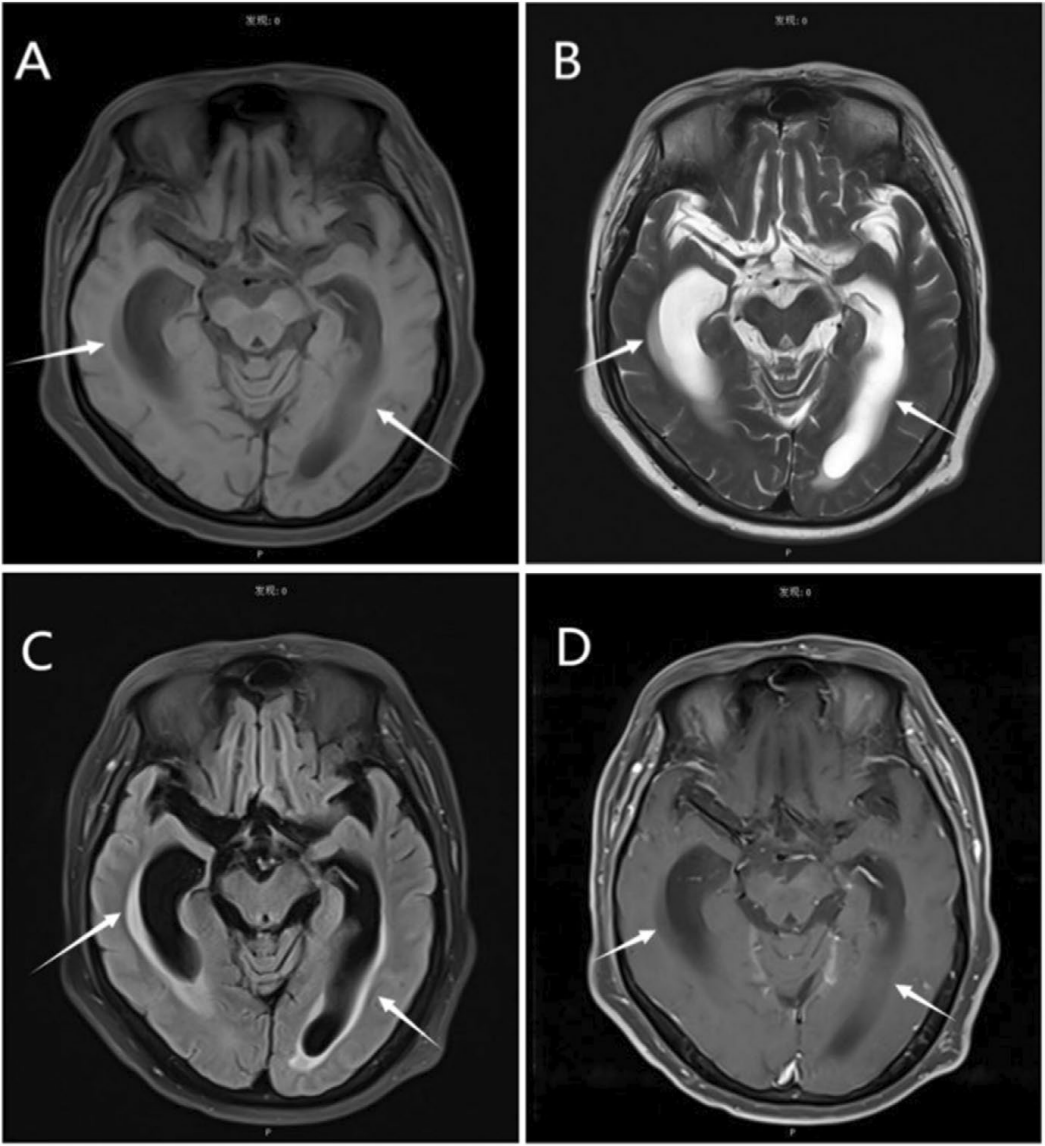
(A) T1‐weighted images (T1WI) showed mild dilatation of the ventricular system of the brain and decreased signal changes in the surrounding cerebral white matter. (B) T2‐weighted imaging (T2WI) represented increased signal. Fluid attenuation inversion recovery (FLAIR) manifested patchy enhancement near the bilateral Lateral ventricle (C), while no relative enhancement was found (D).
